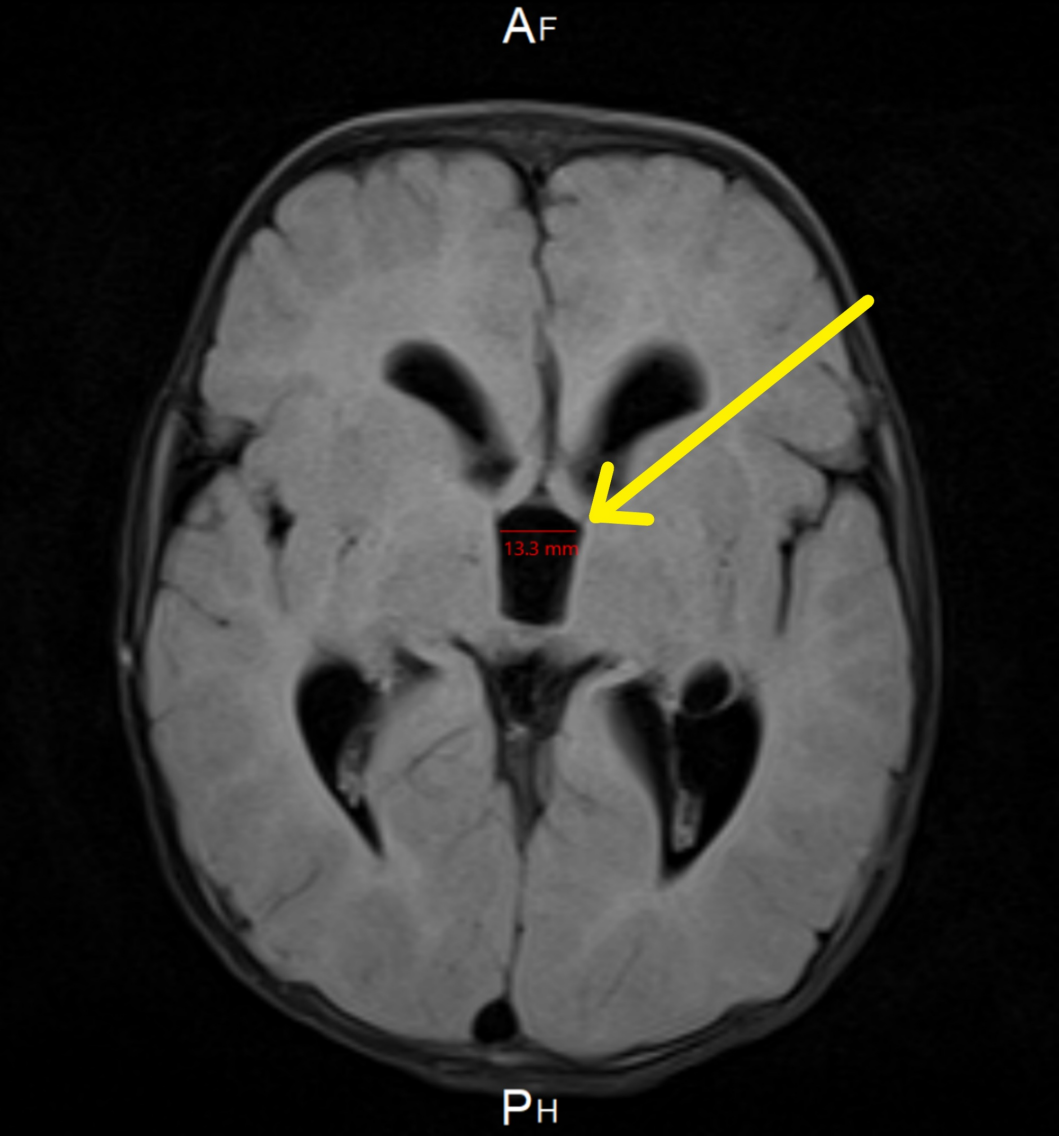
Brain MRI showing dilation of the third ventricle
